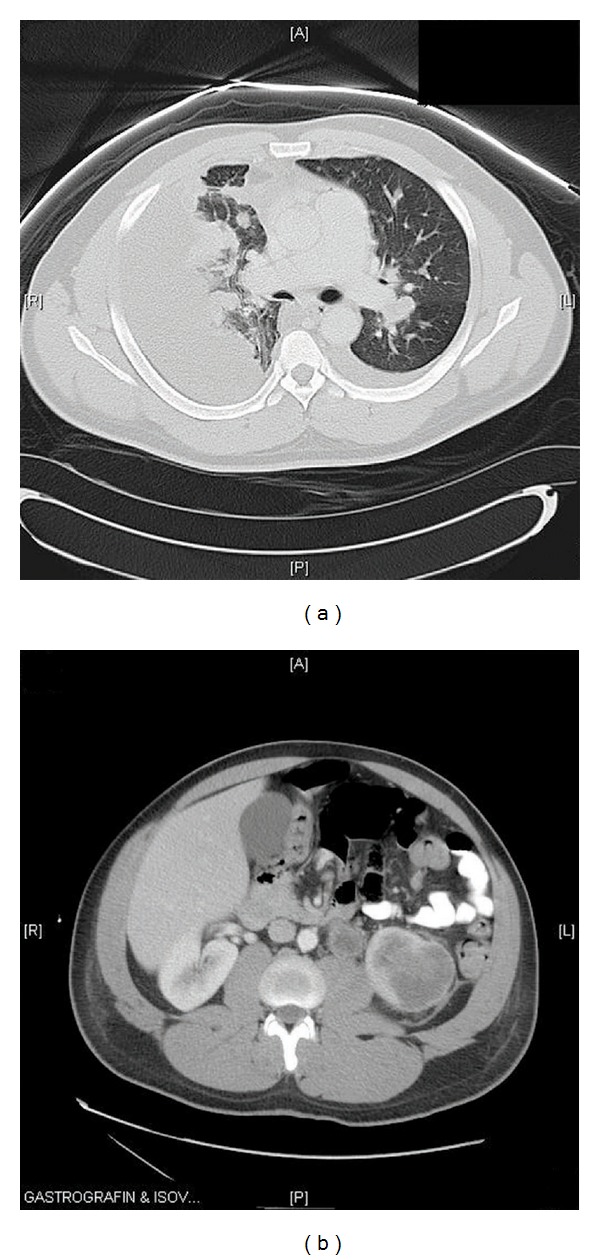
(a) CT scan of the chest in lung windows shows large right pleural effusion, small left pleural effusion, and multiple pulmonary nodules and masses. (b) CT scan of the abdomen shows irregular left lower pole low attenuation cystic renal mass with adjacent lymphadenopathy.
